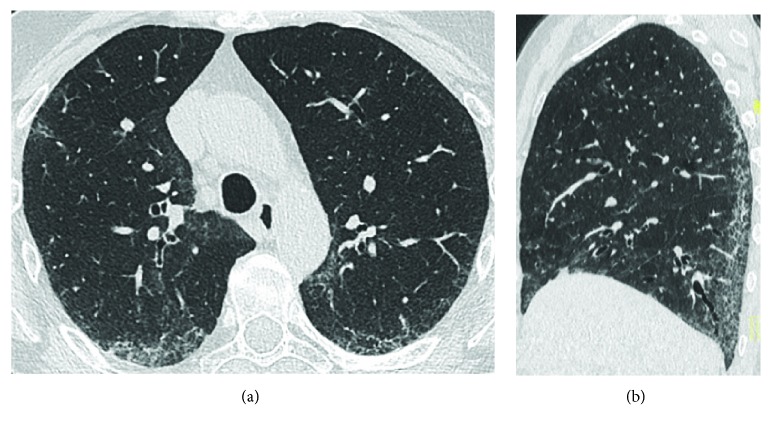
A 67-year-old woman with CD. In both basal posterior segments of the lower lobes, an interlobular-intralobular interstitial thickening associated with ground glass opacities and some traction bronchiectasis and bronchiolectasis is appreciable. A subpleural sparing is associated. These findings are compatible with NSIP. Axial HRCT study (a) and sagittal reconstruction (b).
